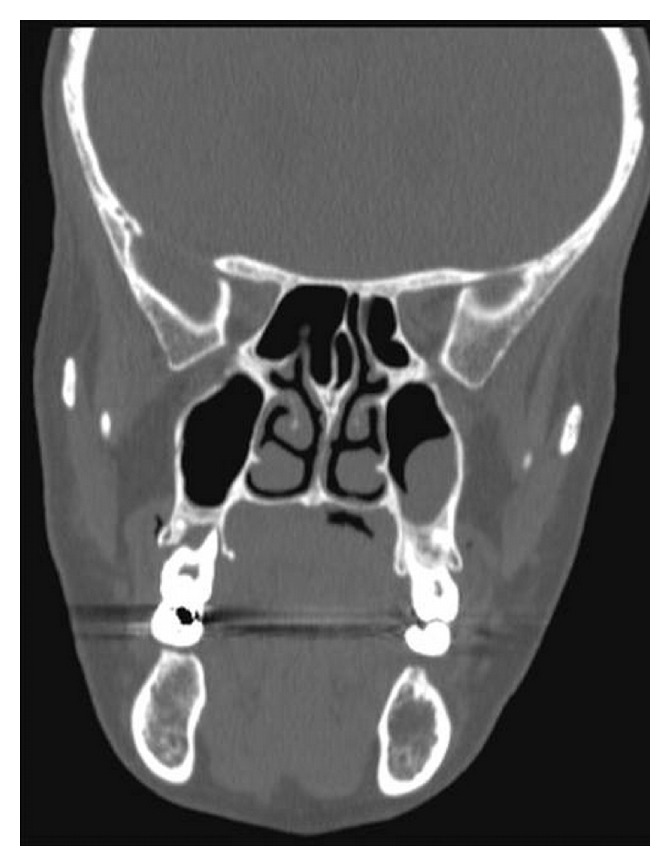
Computed tomography scan showing a mucosal lesion without bone involvement (coronal view).
